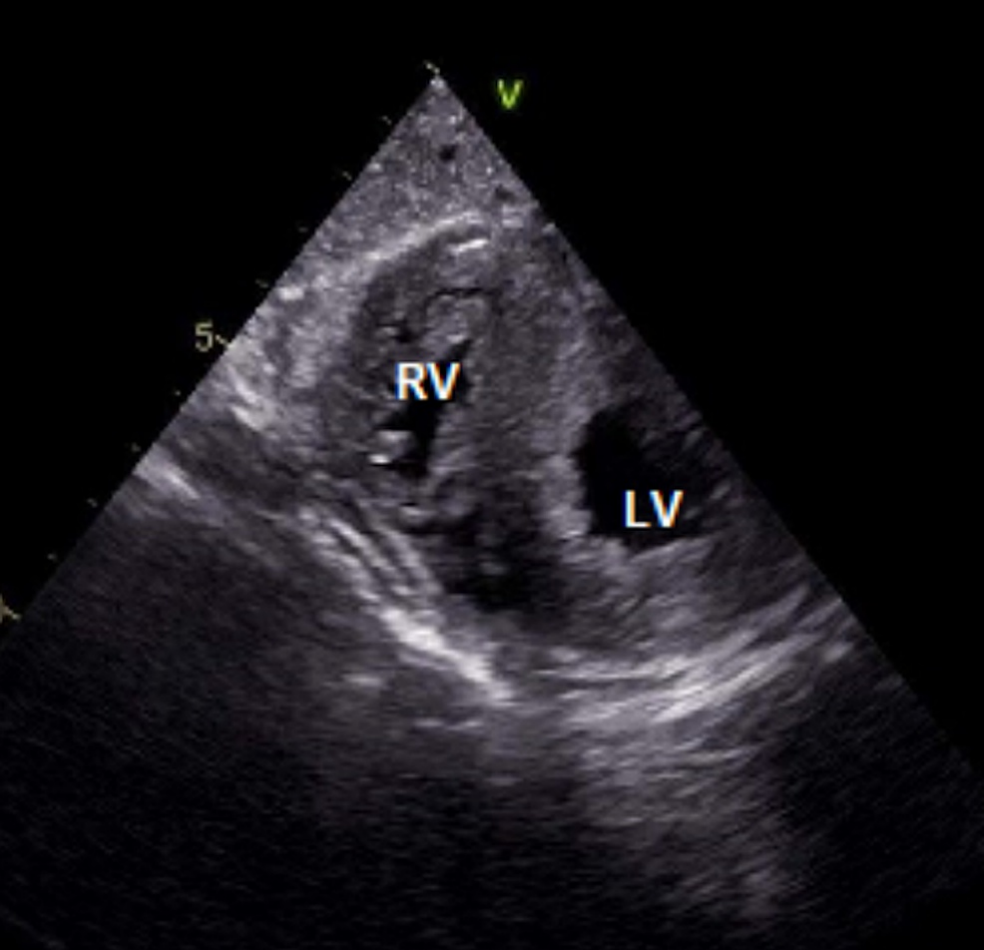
Transesophageal echocardiogram demonstrating ventricular septal hypertrophy from the transverse plane. RV: Right ventricle; LV: Left ventricle
